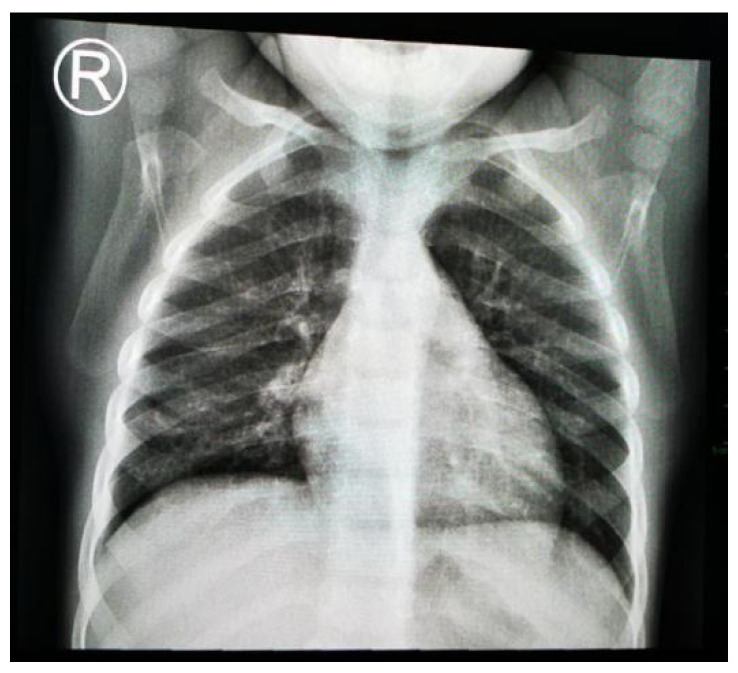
Normal chest X-ray of 2-year-old male child with pulmonary abscess, 3 months post-treatment.
